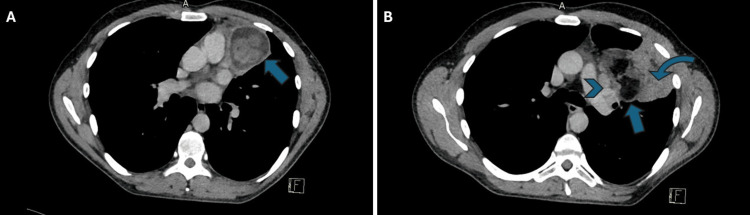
Axial chest computed tomography (CT) images showing mediastinal mass. The lower part of the mass is represented in image (A), which displays a round structure that appears to be cystic (arrow). The upper part of the mass is shown in image (B), which displays elements of fat (arrow), enlarged mediastinal lymph nodes (arrowhead), and consolidated lung tissue (curved arrow).
